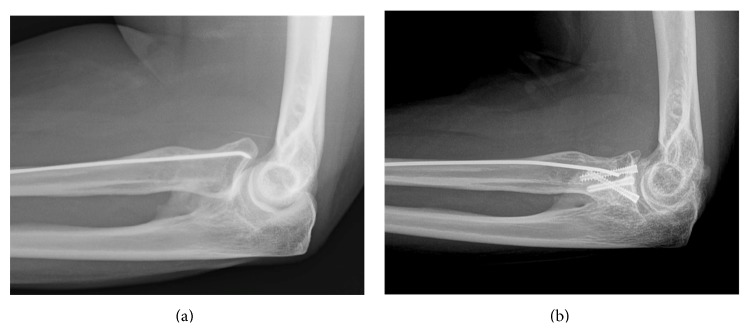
(a) Preoperative lateral X-ray showing the radial neck malunion of 60° dorsal angulation. (b) Postoperative X-ray showing the radial neck osteotomy and fixation.
